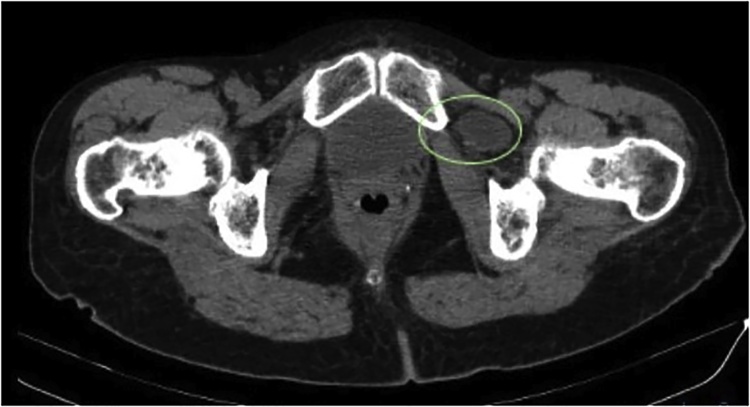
Axial CT imaging demonstrating small bowel within the obturator canal.
